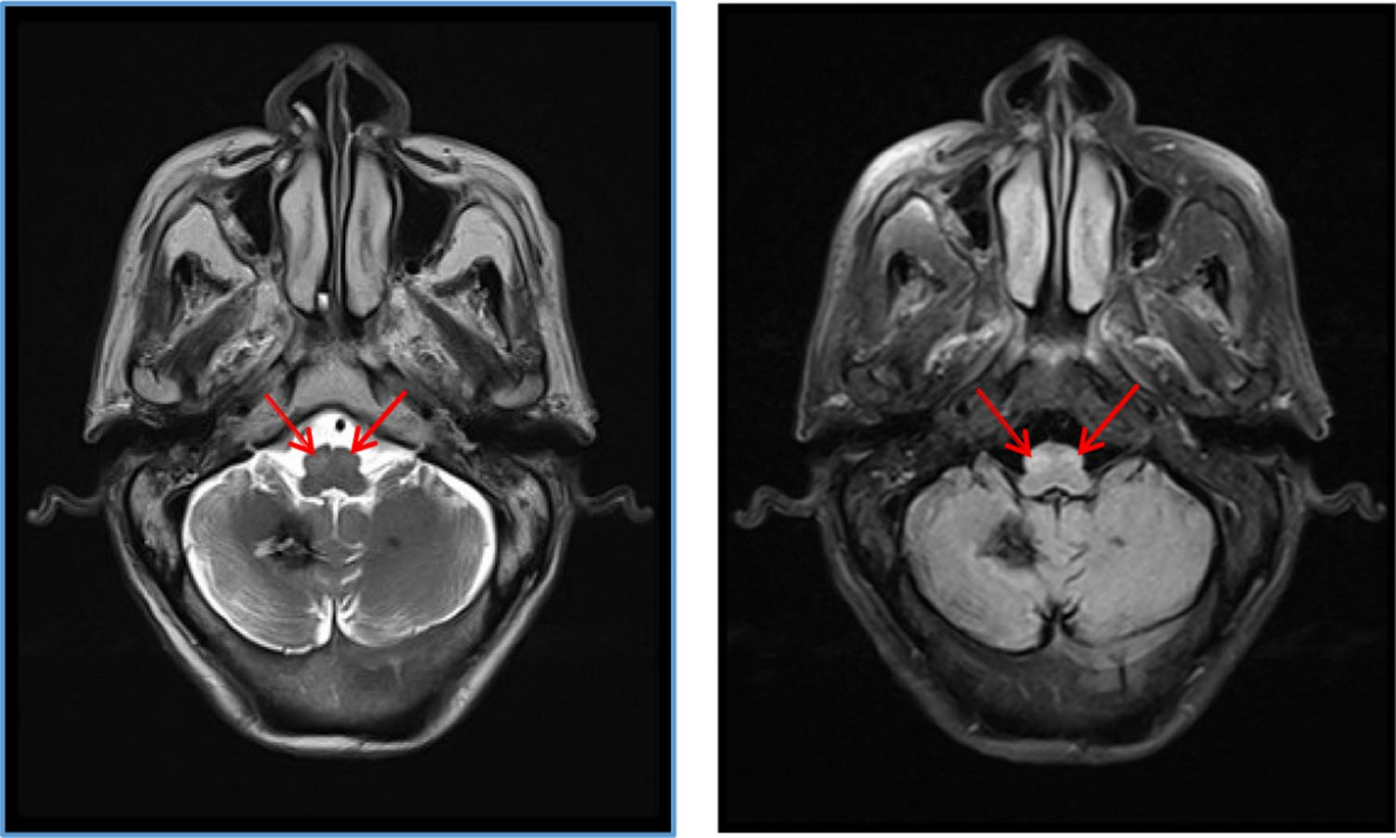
Axial T2 and FLAIR images: long T2 signal shadows were observed in the right cerebellum, and low-density circular shadows were observed around the lesion, with patchy long T2 signal changes in the left cerebellum. The area of the bilateral olivary nucleus was enlarged, with a longer T2-weighted signal change, and the T2 FLAIR image showed an increased signal change, the right ION was obviously larger than the left
